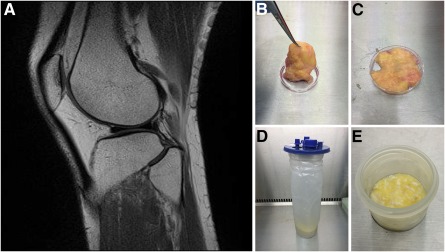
Infrapatellar fat pad location and samples. (A): Sagittal magnetic resonance imaging scan of the knee showing the relationship of the infrapatellar fat pad (IFP; white) to the articular cartilage (black). (B, C): Excised IFP from a patient undergoing knee arthroplasty (B) has the fat removed from the fibrous tissue (C). (D, E): The arthroscopically harvested fat pad (D) was separated from the irrigation fluid before enzymatic digestion (E).
